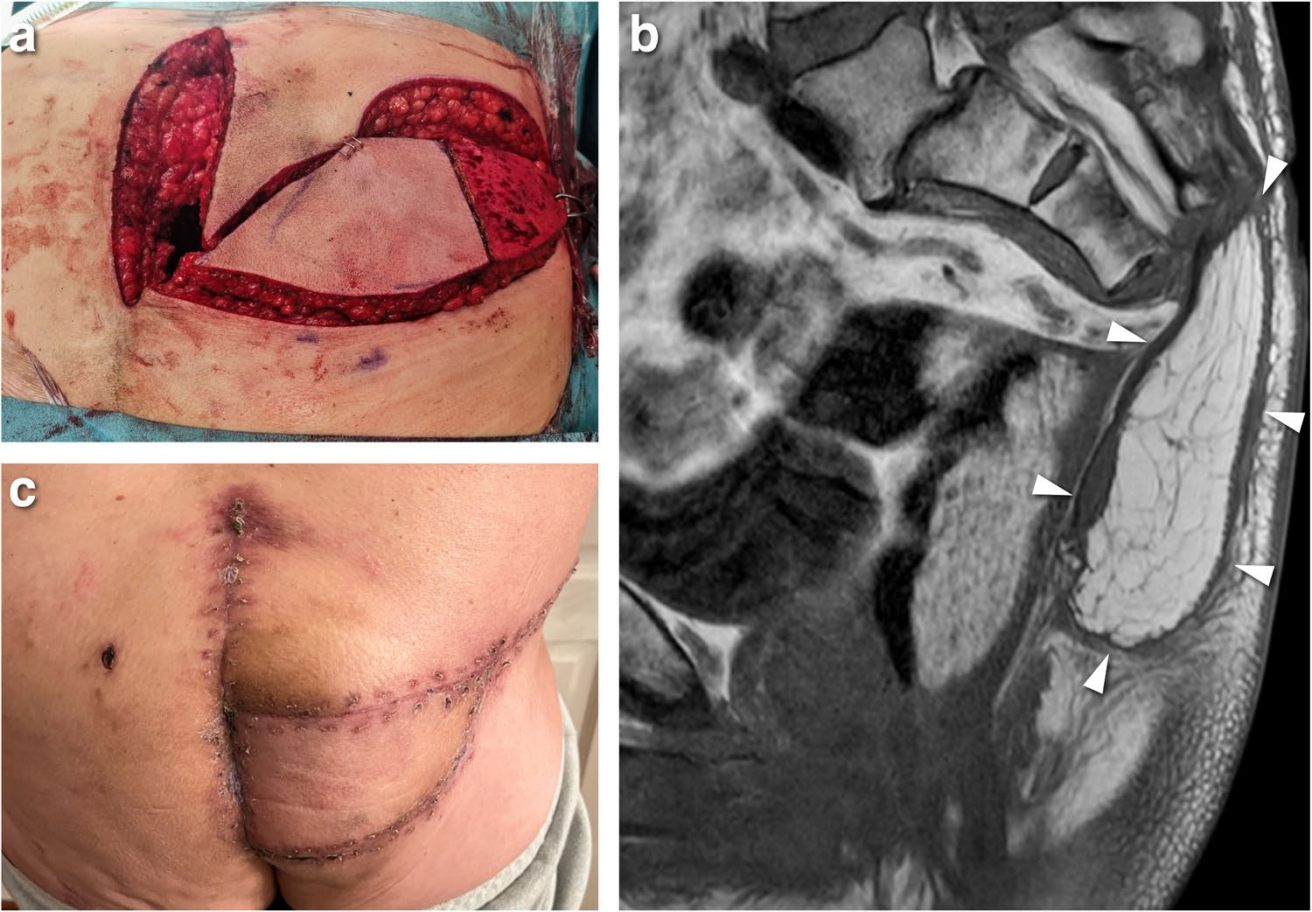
Preoperative and postoperative images. a Preoperative photograph during the soft tissue reconstruction using a partially de-epithelialized superior gluteal artery perforator (SGAP) flap. b Postoperative sagittal non-contrast T1-weighted image shows sacrectomy below the S2 level and soft tissue reconstruction using the SGAP flap (arrowheads). c Clinical image 6 weeks postoperatively showing uneventful healing of the SGAP flap
